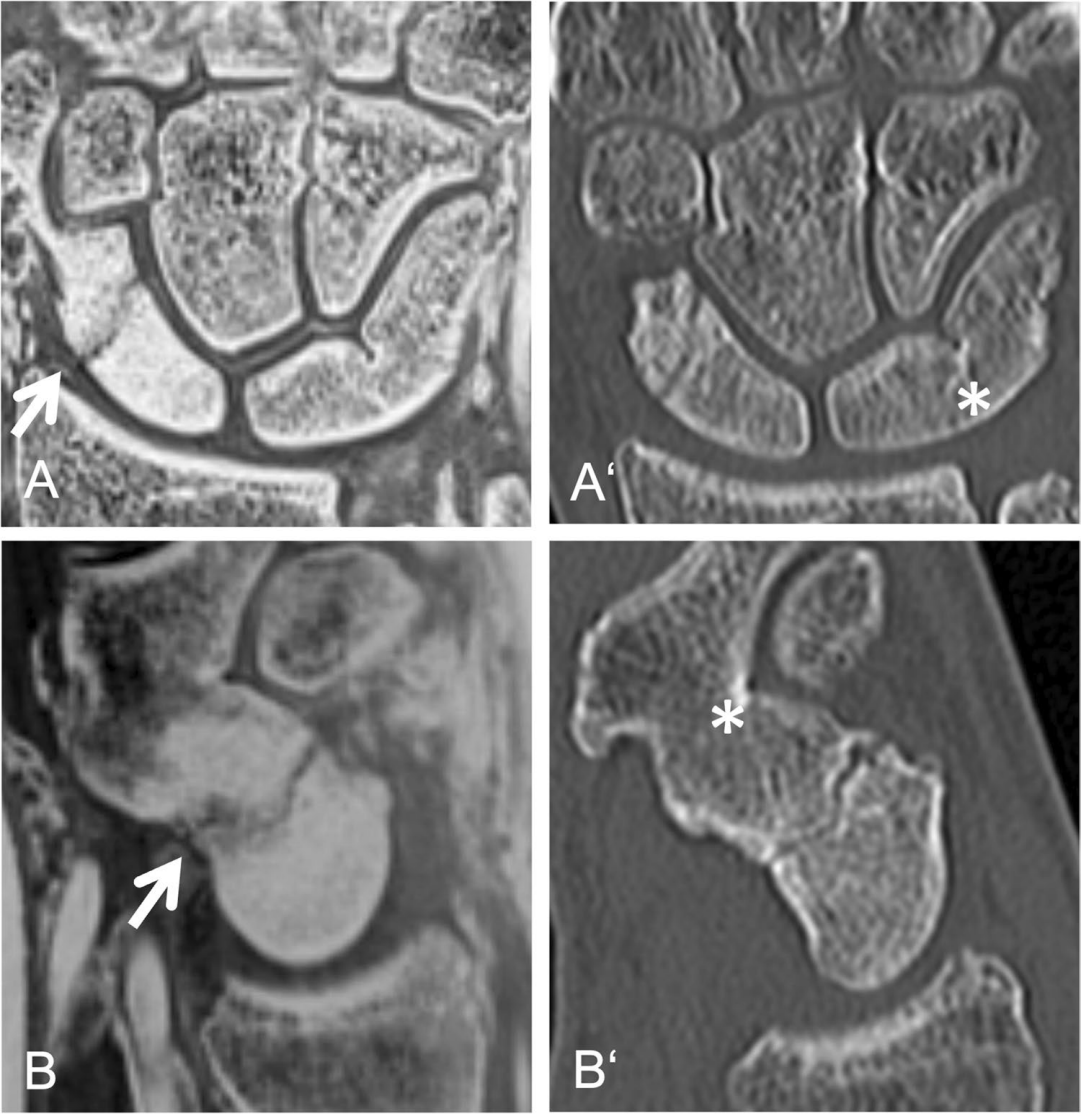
Twenty-three-year-old patient with an acute traumatic scaphoid fracture (arrow). Multiplanar reconstruction in coronal (A, A‘) and parasagittal plane (B, B‘) based on an inverted 3D T1GRE sequence (A, B) and a computed tomography (CT) (A‘, B‘) of a. Note, the bright homogenous oedema adjacent to the fracture line in the CT-like image, not visible on CT. In addition, the patient shows an osseous lunotriquetral and a scaphotrapezial coalition (asterisk (*)). 3D T1GRE, 3-dimensional T1-weighted gradient-echo; CT, computed tomography
